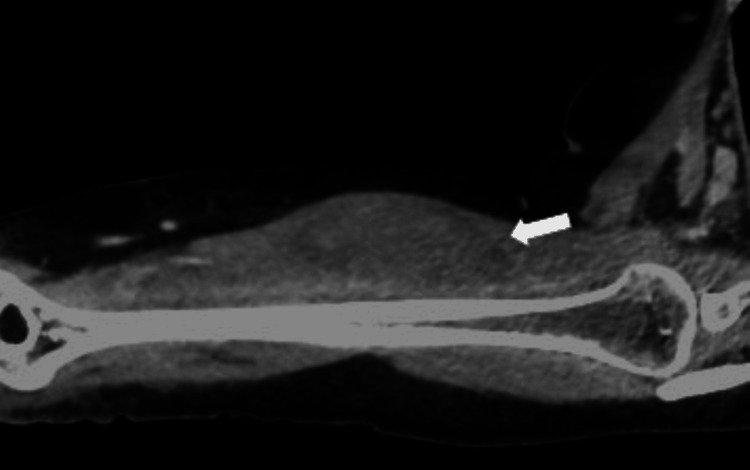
Selected sagittal oblique CT image of the biceps muscle The CT image in the venous phase demonstrates a well-defined hypoattenuating intramuscular collection (white arrow) within the biceps muscle.
